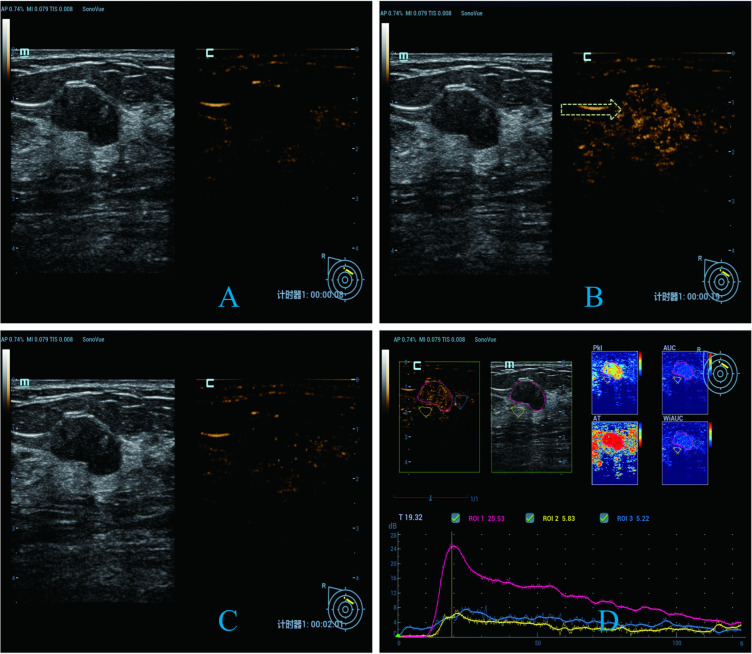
Contrast-enhanced ultrasound (CEUS) images and time–intensity curve (TIC) analysis (A–D). (A) Early wash-in phase. (B) Peak enhancement phase, showing heterogeneous enhancement with linear hyperenhancing structures and hypo- to non-enhancing areas. (C) Late phase, showing contrast washout. (D) Time–intensity curves of the lesion and adjacent glandular tissues. Green dashed arrows indicate linear hyperenhancing areas within the lesion.
